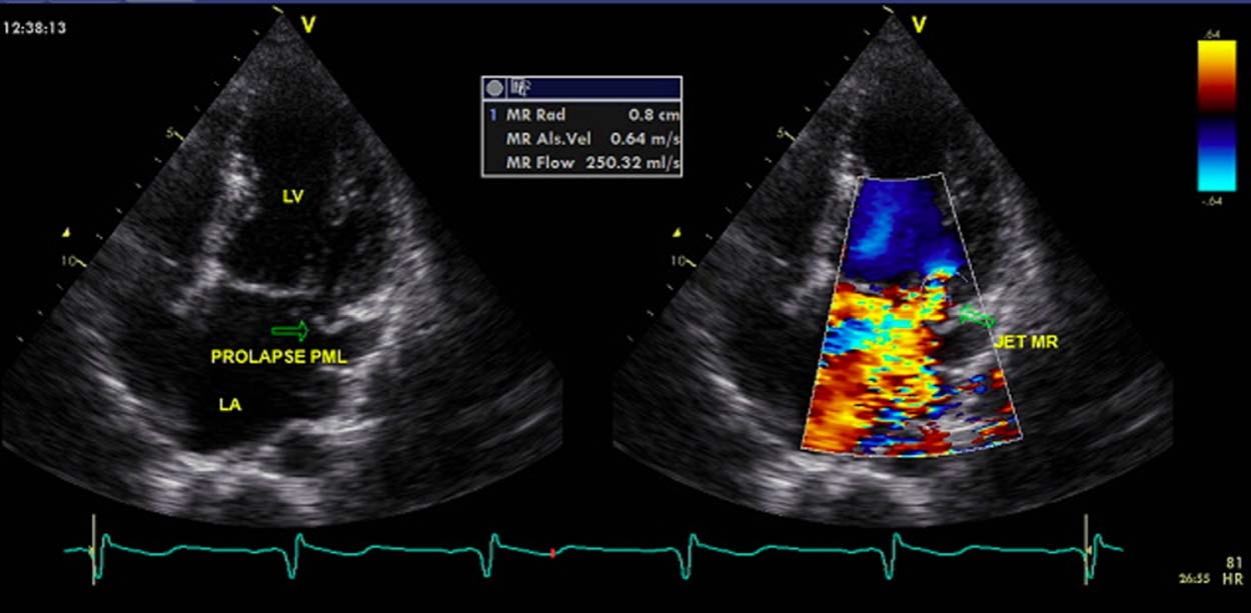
Patient 2 echocardiography. Echocardiography showed left ventricle, preserved ejection fraction, and severe MR with PML prolapse.
